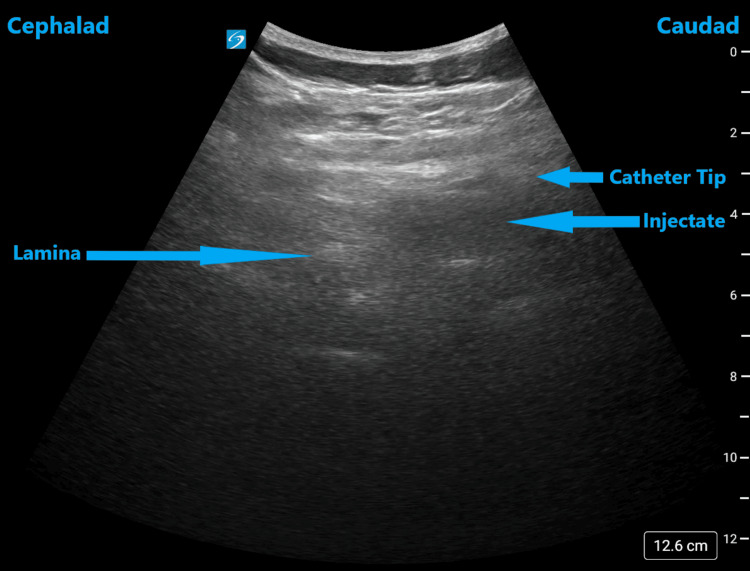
Ultrasound image of the thoracic spine in parasagittal oblique view demonstrating the catheter outside the epidural space
